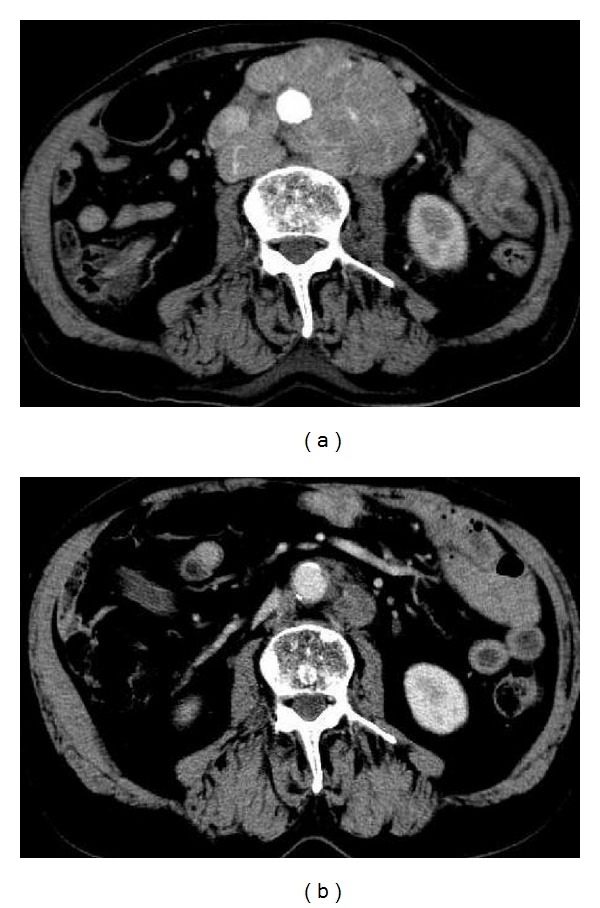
Abdomen computed tomography scans during treatment. (a) Prior to hormonal therapy, showing para-aortic and pelvic lymph node enlargement. (b) Six months after initiation of hormonal therapy, lymph node size decreased to more than 40%.
